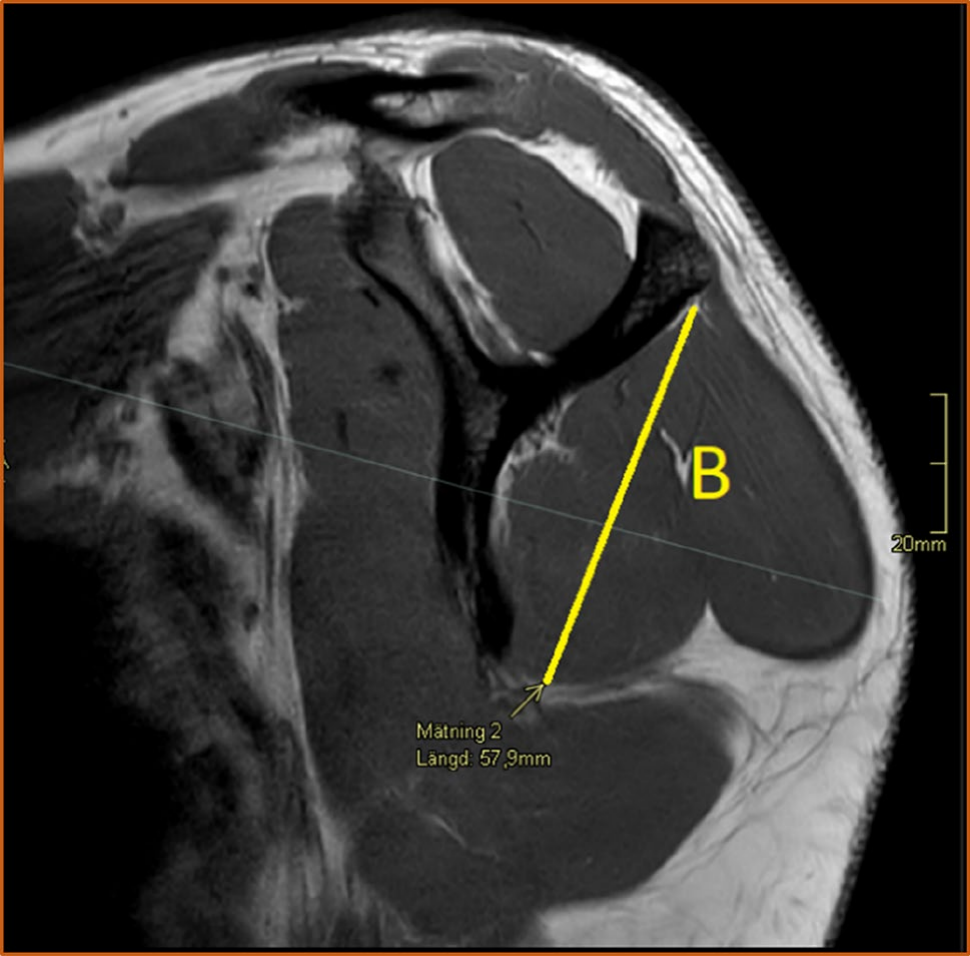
The craniocaudal diameter (B) of the infraspinatus muscle measured in the sagittal view. T2-weighted image, turbo spin echo. Sagittal section
